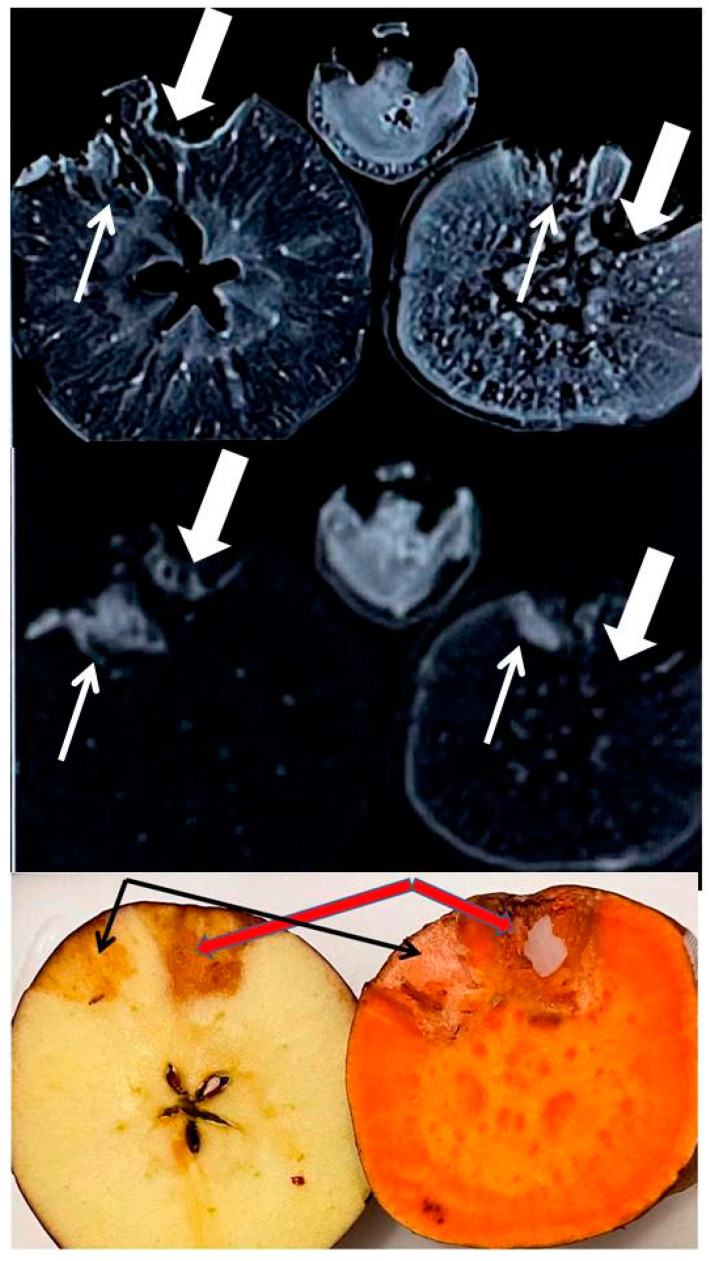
High Field (3T) MR images of the mineral-rich apple (left) and sweet potato (right), each with two carved wells infused five days prior with iodinated contrasts Omnipaque (240, thin arrow and 350, thick arrow). Also shown is the image of a banana at the superior/middle aspect.
